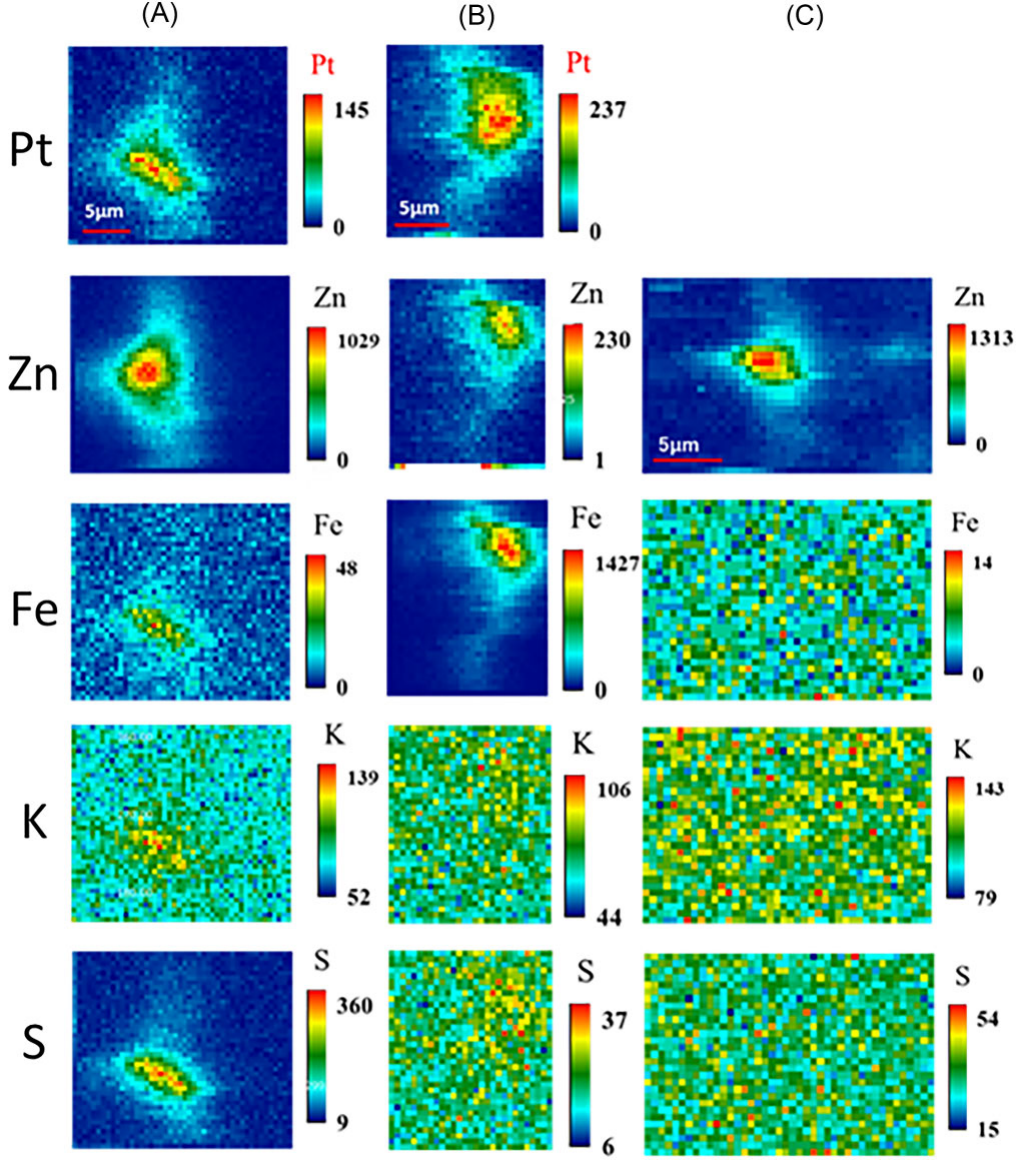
Elemental mapping in unicellular alga obtained by synchrotron radiation X-ray microbeam. (A) *In vivo* cell with platinum addition, (B) lyophilized cell with platinum addition, and (C) control cell. The concentration of the platinum solution added was 100 ppm, and the addition time was 24 hours, under light-shielded conditions. X-ray energy; 12.5 keV (A and B), 10 keV (C). X-ray beam size; 0.8 μm (V)×1 μm (H), step size; 0.5 μm (V)×0.5 μm (H), and measurement time; 2 s/point. Imaging area 23 μm(V)×20.5 μm(H)(A), 14.5 μm(V)×17.5 μm(H)(B), and 21 μm(V)×14.5 μm(H)(C).
